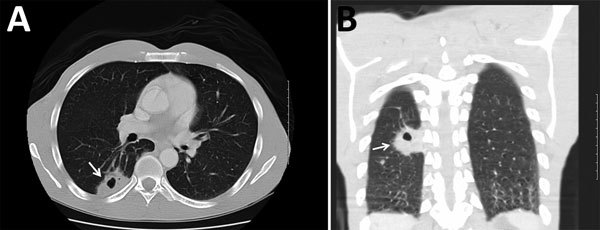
Computed tomography images of the chest of an HIV-infected 47-year-old man (case-patient 3) with *Cryptococcus gattii* complex infection, southeastern United States. Transverse (A) and frontal (B) views without intravenous contrast showed a mass (arrows) (4.0 cm × 2.5 cm) that had central cavitation posteriorly in the right lower lobe abutting the pleural surface. The central cavitary portion of this lesion had a maximum length of ≈1.3 cm and no evidence of fluid level or internal soft tissues
